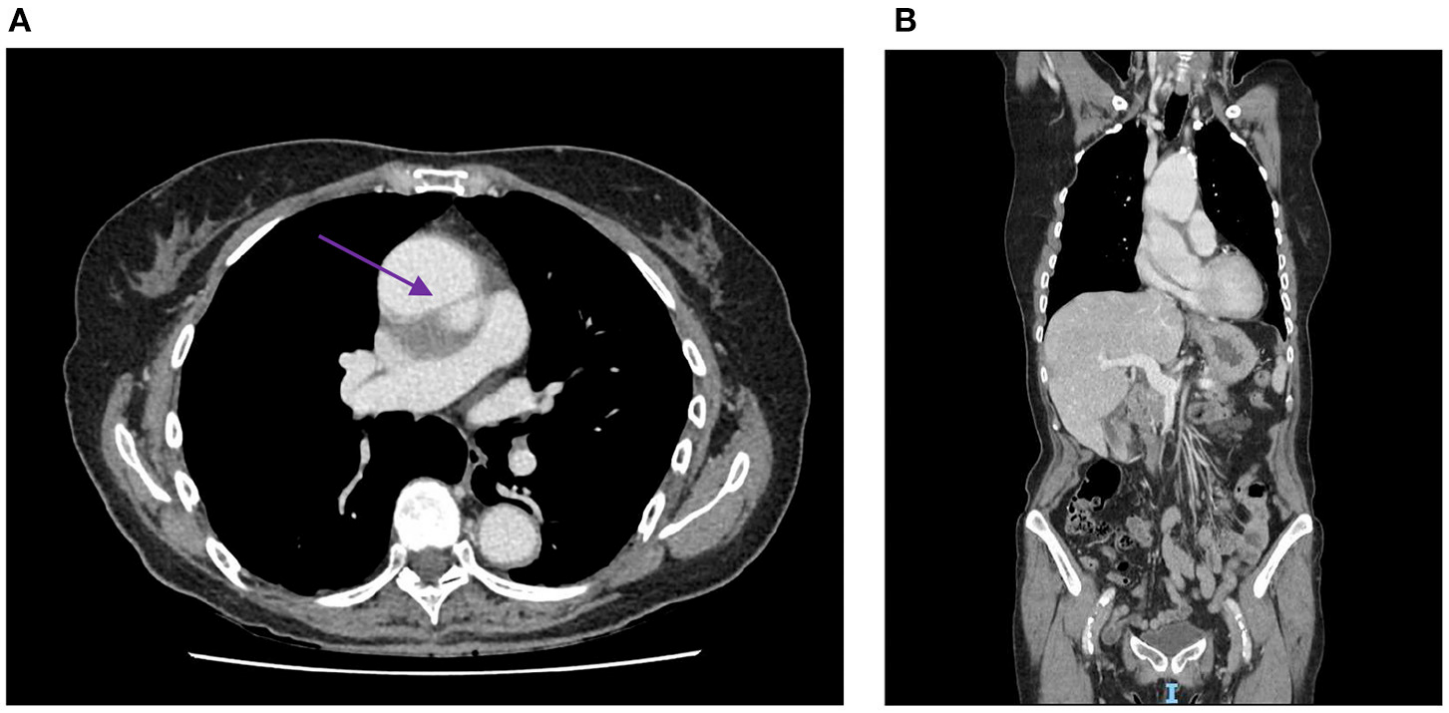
(A) Contrast medium CT scan showing an aneurysm of the ascending aorta (53 × 54 mm) with a tear of the intima, creating a thick false lumen (4 mm), which is indicated by the purple arrow. (B) Sagittal section of the contrast medium CT scan, which also shows the aneurysm and thick tear of the intima. CT, computed tomography.
